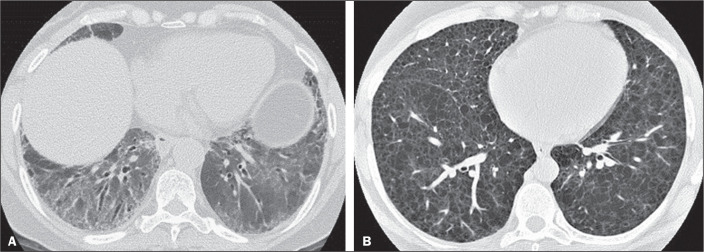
Examples of predominant HRCT imaging patterns. A: A 68-year-old patient
